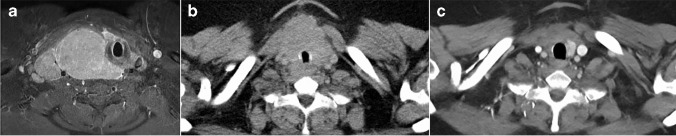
a MRI imaging showing a well-circumscribed pronounced mass occupying the right cervical region with infiltration of the thyroid bed and displacement of the trachea (corresponding to the NUT carcinoma). b CT imaging showing significant disease response after completion of definitive radiochemotherapy. c CT imaging on the last follow-up examination without signs of locoregional recurrence
